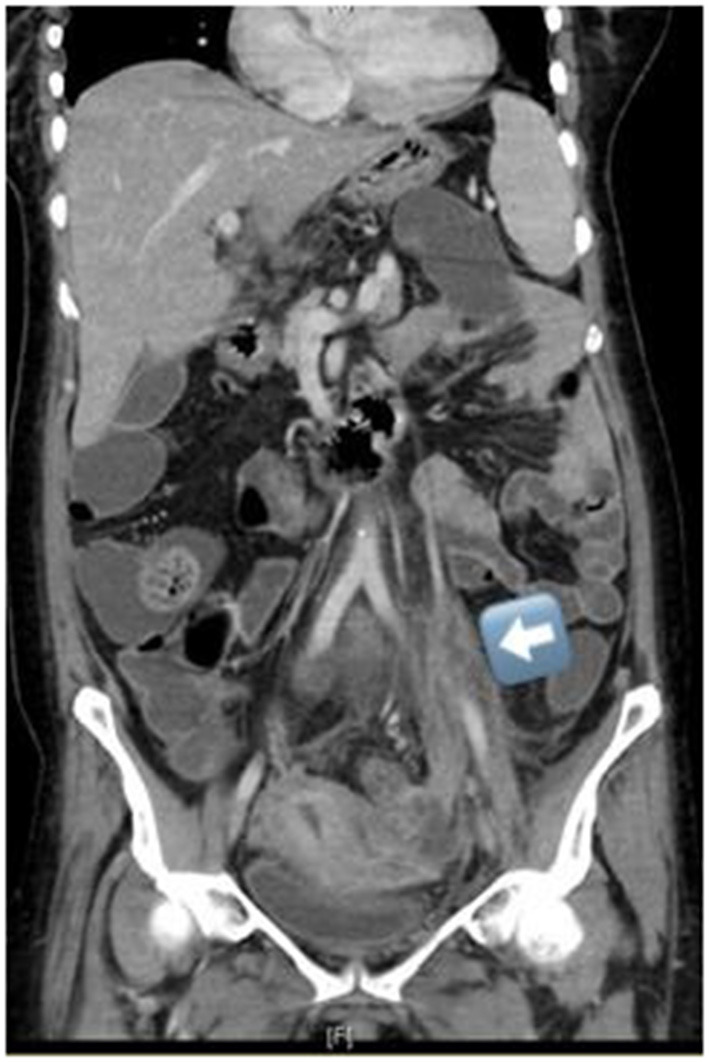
CT abdomen with contrast exhibiting left ovarian vein thrombosis.
